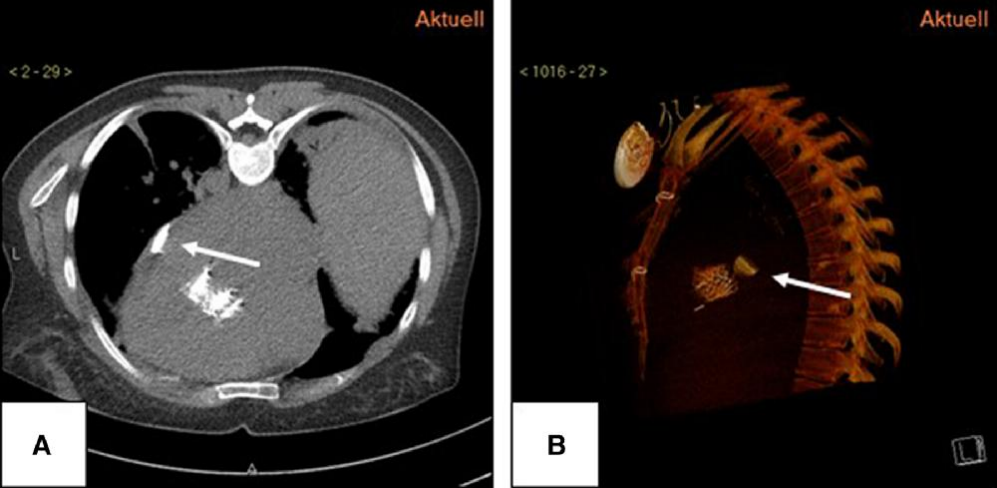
Chest CT taken in prone position in transverse plane (*A*) and sagittal plane (*B*) showing the tilt (white arrow) in the antero-lateral left atrium.
